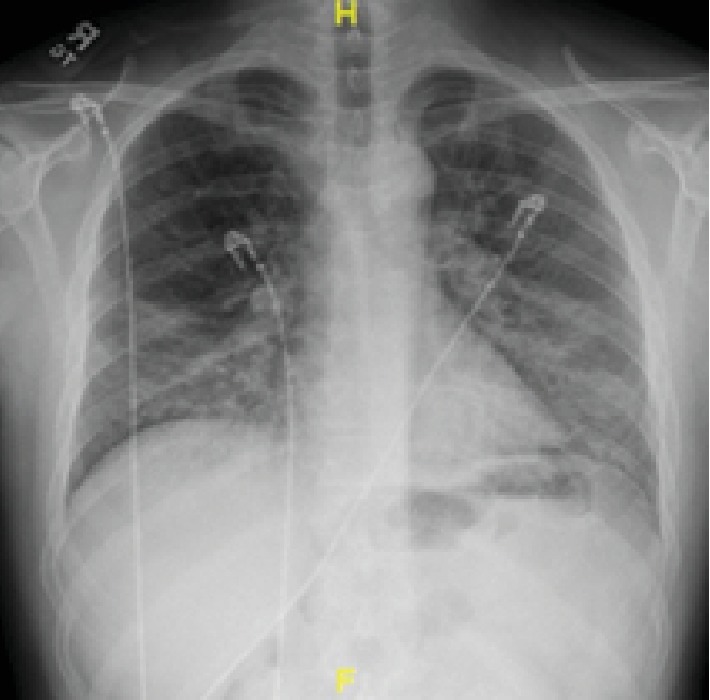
Initial chest X-ray showing bilateral patchy infiltrates with lower lobe predominance.
